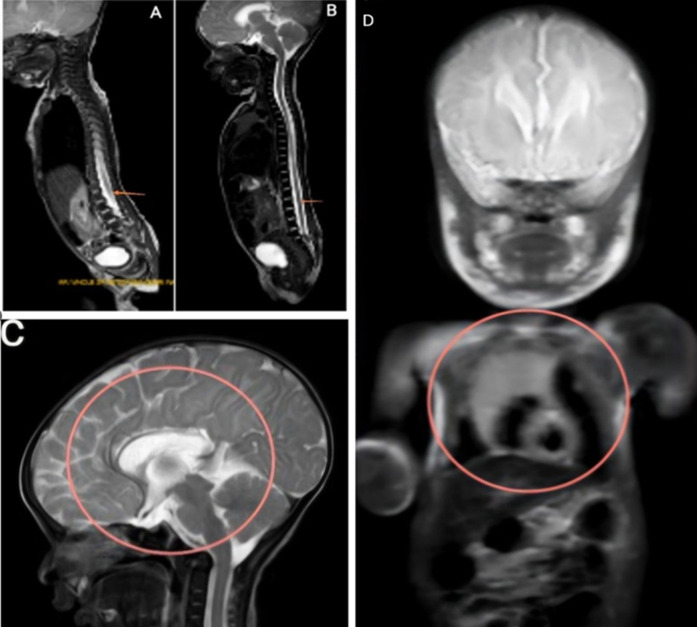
A) preoperative MRI of the whole spine showing a defect in the posterior elements of the sacral superior endplate; B) postoperative MRI of the whole spine showing hypointensity in the cord; C) brain MRI showing severe thinning of the genu and body of the corpus callosum; D) MRI of the whole body showing dextrocardia without situs inversus
